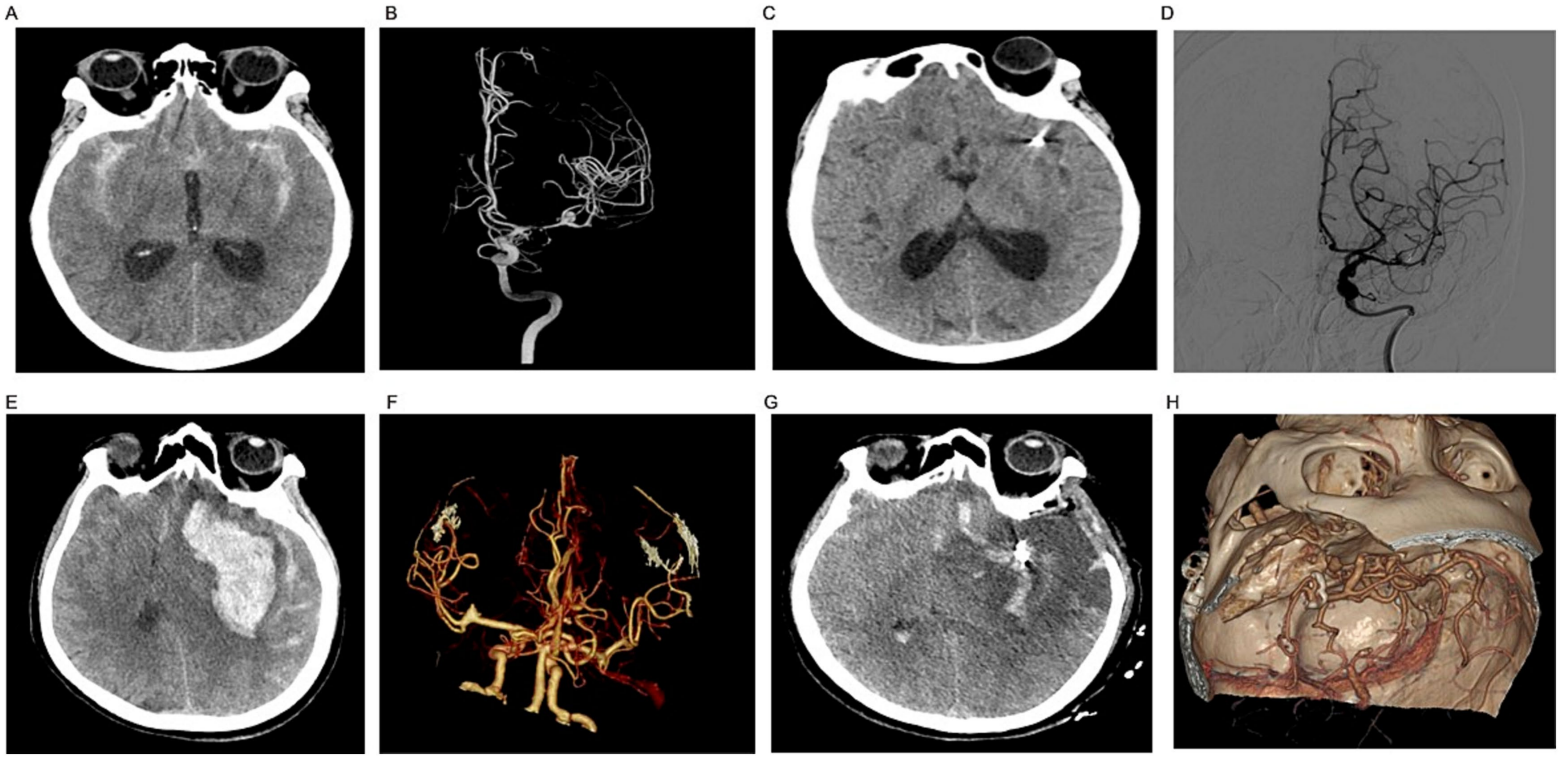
(A) Ruptured aneurysm of the left middle cerebral artery with subarachnoid hemorrhage. (B) DSA showing left middle cerebral artery aneurysm. (C) Post-embolization CT of left middle cerebral artery aneurysm. (D) Post-embolization angiogram of left middle cerebral artery aneurysm. (E) Left cerebral middle artery aneurysm rupture with subarachnoid hemorrhage and temporal lobe hematoma. (F) Left cerebral middle artery aneurysm CTA imaging. (G) Post-clipping of left cerebral middle artery aneurysm and clearance of temporal lobe hematoma. (H) Post-clipping of left cerebral middle artery aneurysm.
